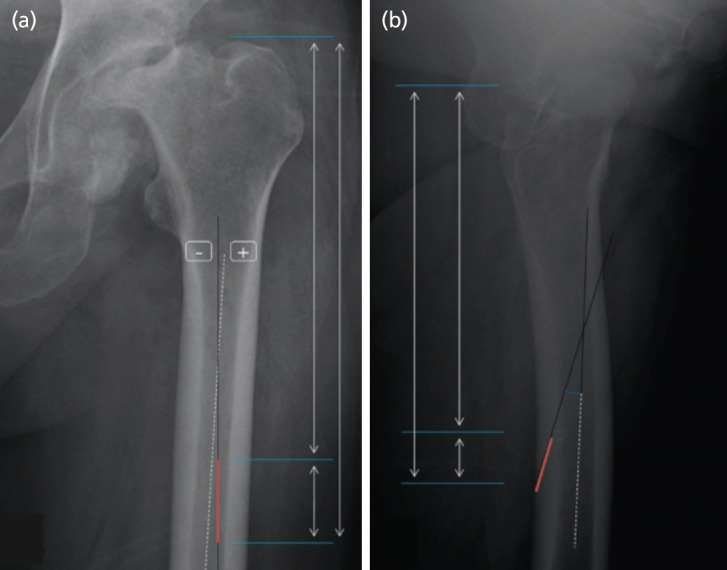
Radiographic measurement of the nutrient artery canal. (a) Anteroposterior view. (Bold red line: nutrient artery canal, Dotted white line: anatomical axis of femur, +: positive value of angle, -: negative value of angle, Inner superior white arrow line: distance from the tip of the greater trochanter to the proximal end of nutrient artery canal, Inner inferior white arrow line: length of nutrient artery canal, Outer white arrow line: distance from the tip of the greater trochanter to the distal end of nutrient artery canal). (b) Translateral view. (Bold red line: nutrient artery canal, Dotted white line: central line of femur. The rest indicate the same things of anteroposterior view).
